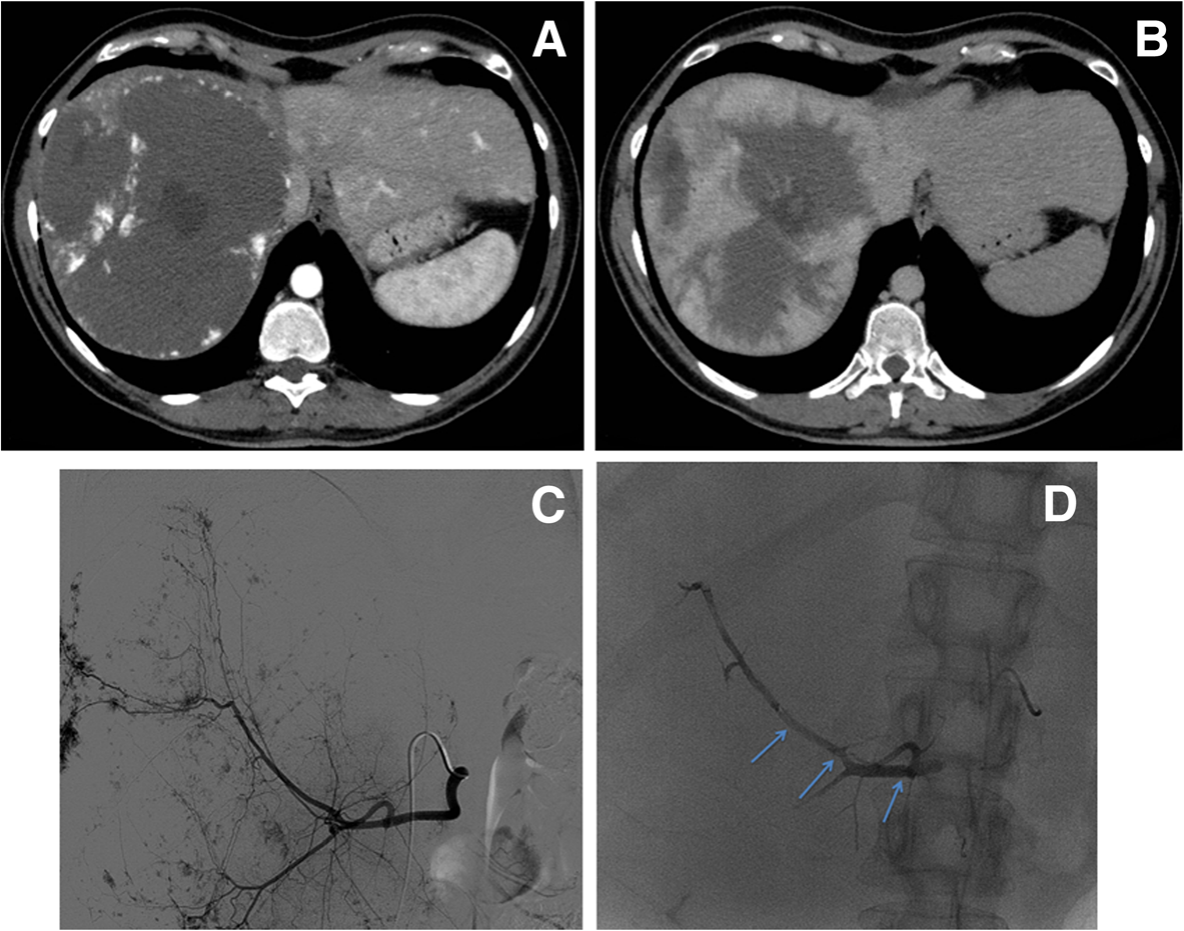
Preoperative embolization. a Contrast-enhanced CT in arterial phase of a hepatic giant hemangioma. b Contrast-enhanced CT in late phase. c Diagnostic DSA of the hepatic artery. d Final angiographic control after preoperative embolization using PVA particles and Squid (light blue arrows)
